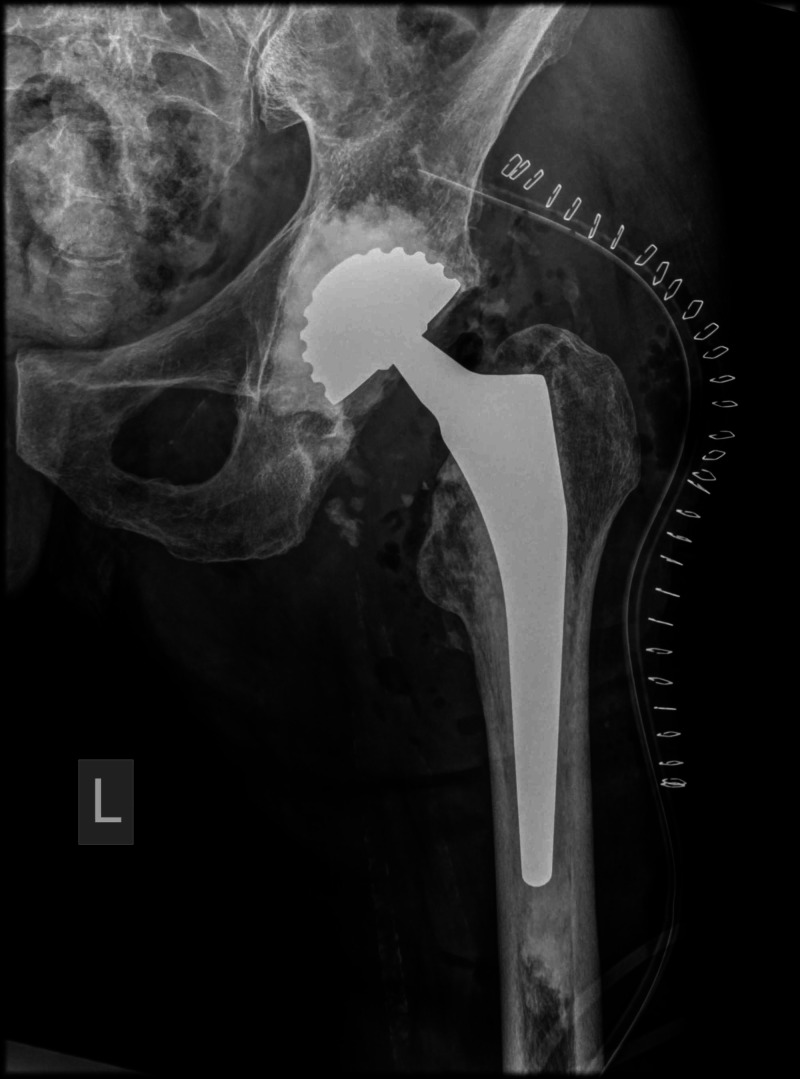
Postoperative anteroposterior left hip radiograph demonstrating re-revision of cemented constrained liner to a cemented dual-mobility cup of the left total hip arthroplasty
